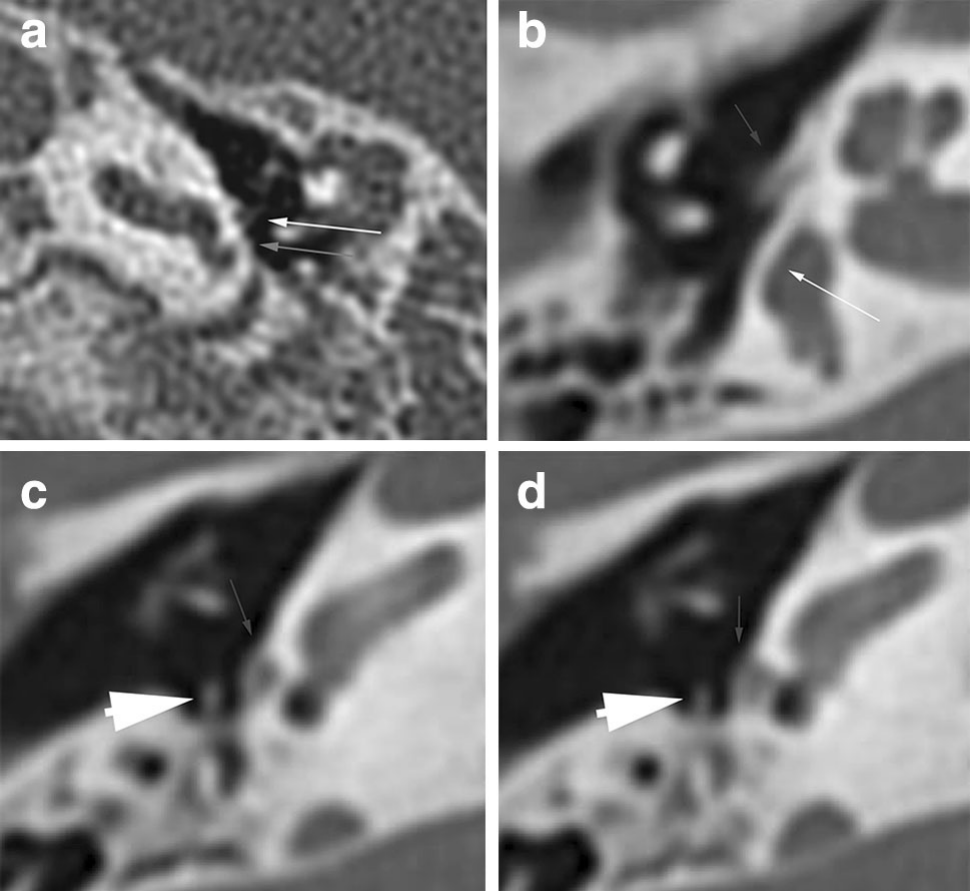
Examples of window stenosis. a Axial CT image showing stenosis of the round window niche (*grey arrow*) in a left ear. Note also dysplastic stapes on the promontory (*white arrow*). b–d Axial CT images showing atresia of the oval window (*thin arrow*), aberrant course of the facial nerve crossing the round window (*arrowhead*) and a dysplastic stapes positioned at the sinus tympani (*thick arrow*). Note aplasia of the semicircular canals
